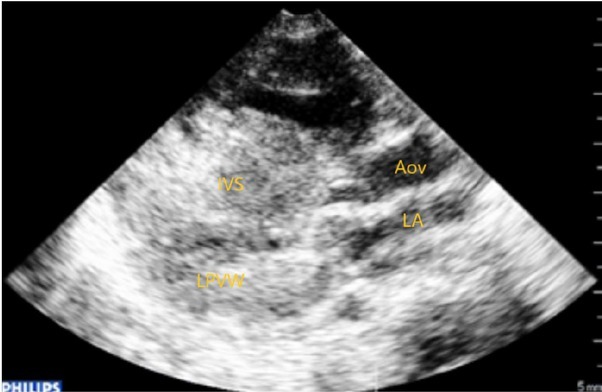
Transthoracic echocardiography parasternal long axis view: hypertrophic cardiomyopathy predominantly septal (IVS-interventricular septum, LPW-left posterior ventricular wall, LA-left atrium, AoV-aortic valve)
